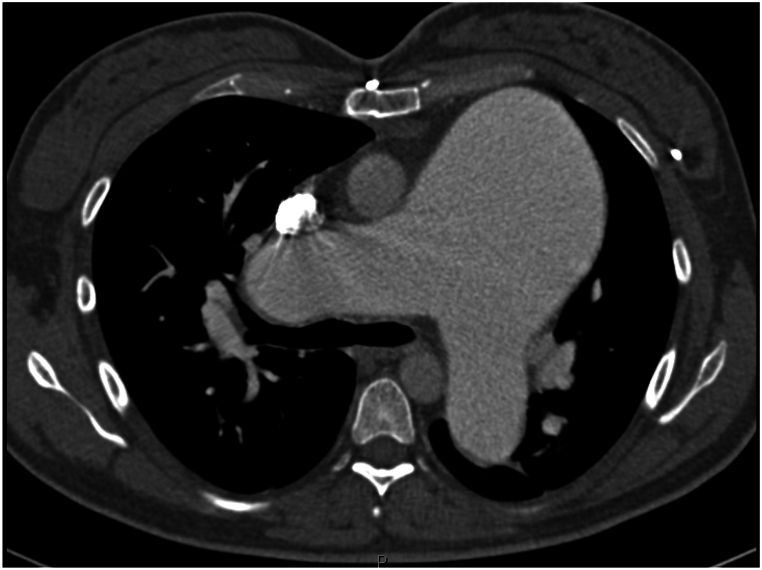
Coronal Computed Tomography (CT) scan of the chest taken in 1997. The image reveals an enlarged main pulmonary artery consistent with severe PAH.
